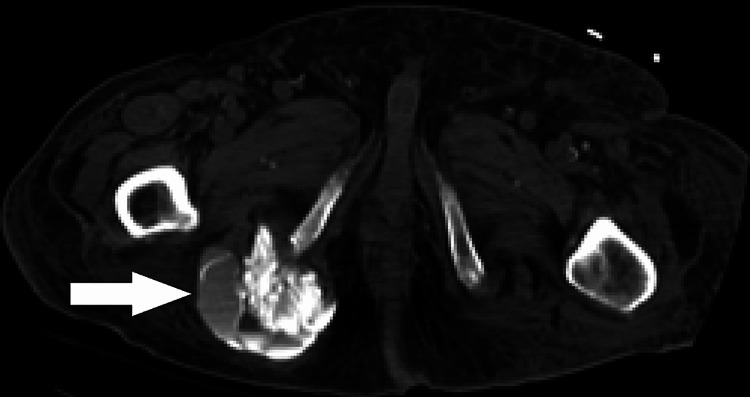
Non-contrast CT of the abdomen and pelvis one year before the current presentation depicting layering hyperdense material in the right pelvic cystic lesion with fluid calcium levels (white arrow), consistent with tumoral calcinosis.
